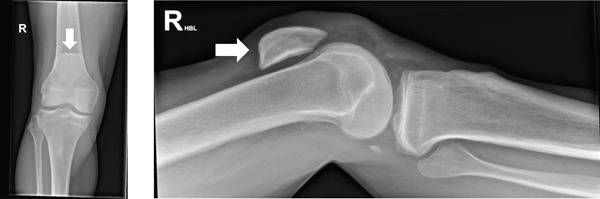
Right knee radiograph demonstrating superior displacement of the patella (arrow), consistent with patella alta and disruption of the extensor mechanism, in keeping with patellar tendon rupture.
